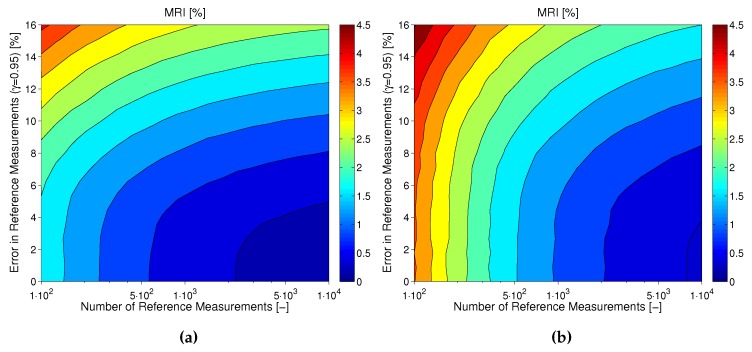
Comparison in terms of MRI for a CGM with (a) relatively good performance (CGM2) vs. (b) a CGM with worse performance (CGM3).
